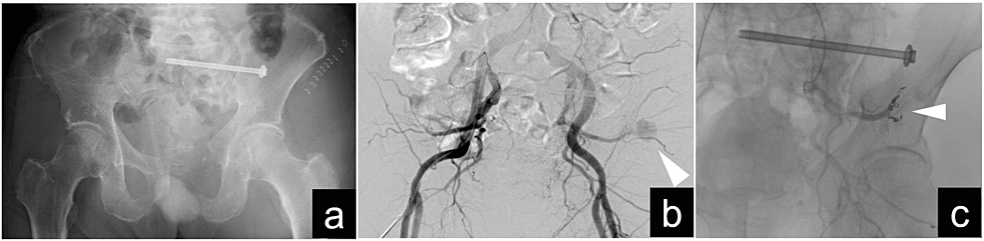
Postoperative radiograph and intravascular radiology imaging. (a) Anteroposterior radiograph after open reduction and internal fixation utilizing an iliosacral screw. (b) Intravascular radiology imaging captured hemorrhaging from the superior gluteal artery. (c) Intravascular radiology imaging confirmed coil embolization of the hemorrhagic site.
